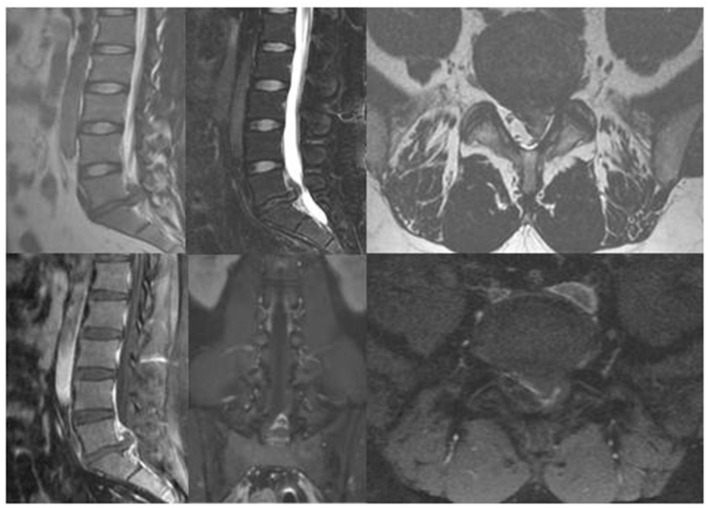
Representative Case: a 28-year-old male patient initially presented at our outpatient clinic in December 2016 with low back pain accompanied by left lower limb numbness and pain for one month, diagnosed with Lumbar Disc Herniation. Lumbar MRI revealed a large extruded disc at the left L5/S1 level, with significant compression of the dural sac. Axial view of contrast-enhanced lumbar MRI showed positive rim enhancement around the herniated nucleus pulposus.
